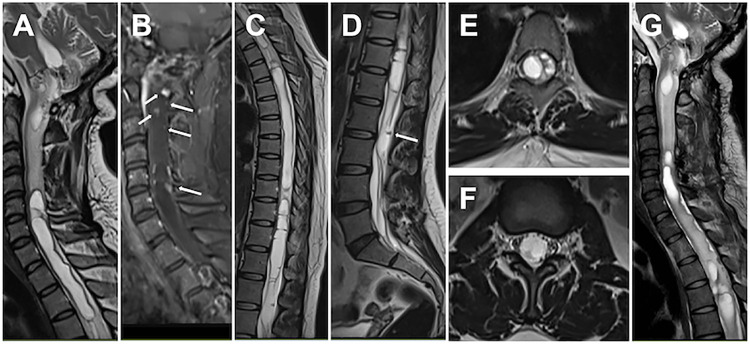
Spinal MRI of Case 3 demonstrating holocord syrinx from medulla oblongata to cone (A–D), with multiple contrast-enhanced intramedullary hemangioblastomas (white arrow). Axial MRI at the T4 and L2–3 levels showing the syrinx cavity in association with focal intramedullary lesions (E,F). Follow-up cervical MRI demonstrating no resolution of the syrinx at the surgically treated level (G).
